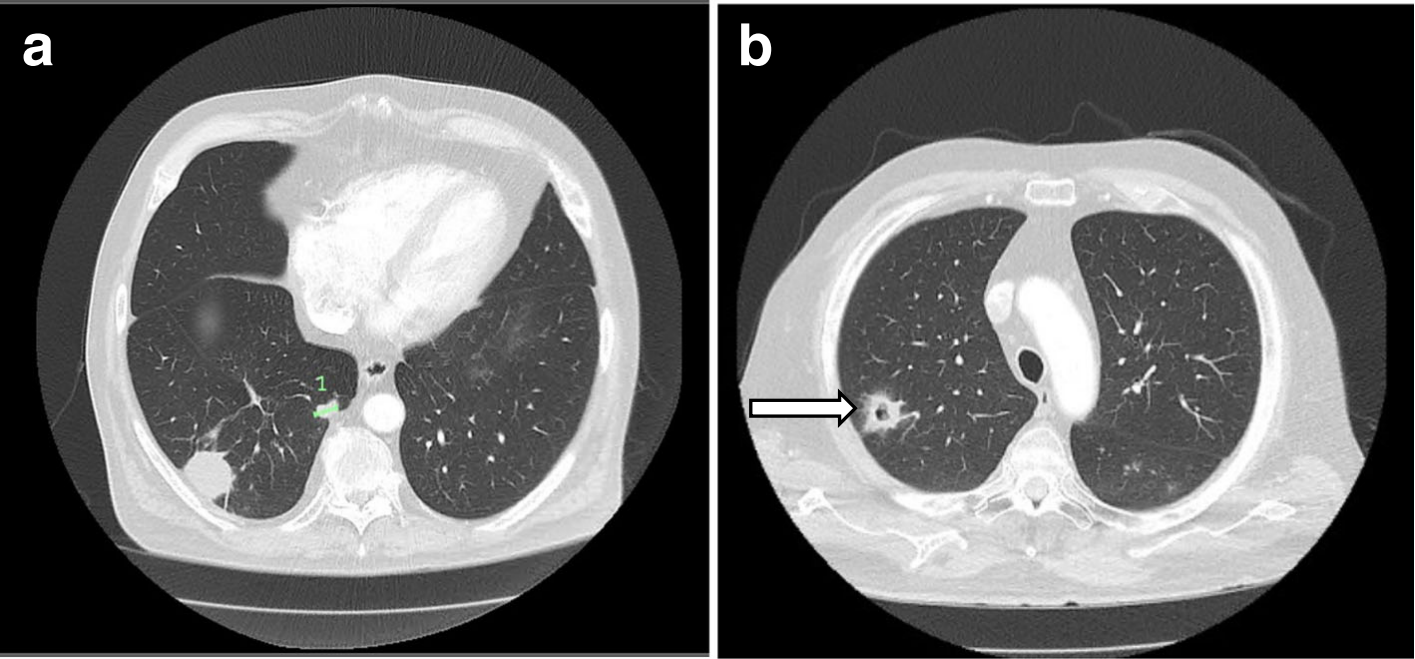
Repeat computed tomographic scans of the chest showing multiple emboli and abscesses despite antibiotics. a A 37-mm opacity in the right lower lobe posteriorly and inferiorly, abutting the pleura and an adjacent 14-mm opacity (*highlighted 1*). b An upper lobe abscess (*arrow*)
